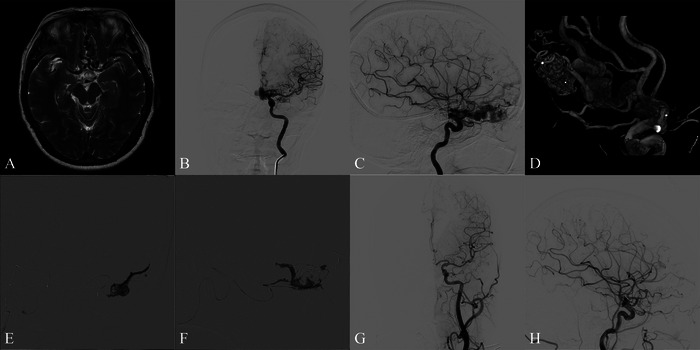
The patient was admitted with headaches. A T2‐weighted MRI revealed an abnormal flow void at the anterior cranial fossa (A). Cerebral angiography was performed, with anteroposterior (B) and lateral (C) views of the left internal carotid artery showing a vascular malformation in the anterior cranial fossa, supplied by branches of the anterior cerebral and ethmoidal arteries. 3D reconstruction clearly visualized the nidus (D), confirming the diagnosis of an arteriovenous malformation (AVM). Onyx‐18 embolization was carried out through multiple arterial feeders (orbitofrontal artery and ethmoidal artery) under fluoroscopic guidance (E, F). Post‐procedure angiography in anteroposterior (G) and lateral (H) views confirmed complete obliteration of the AVM, with preservation of normal branches.
